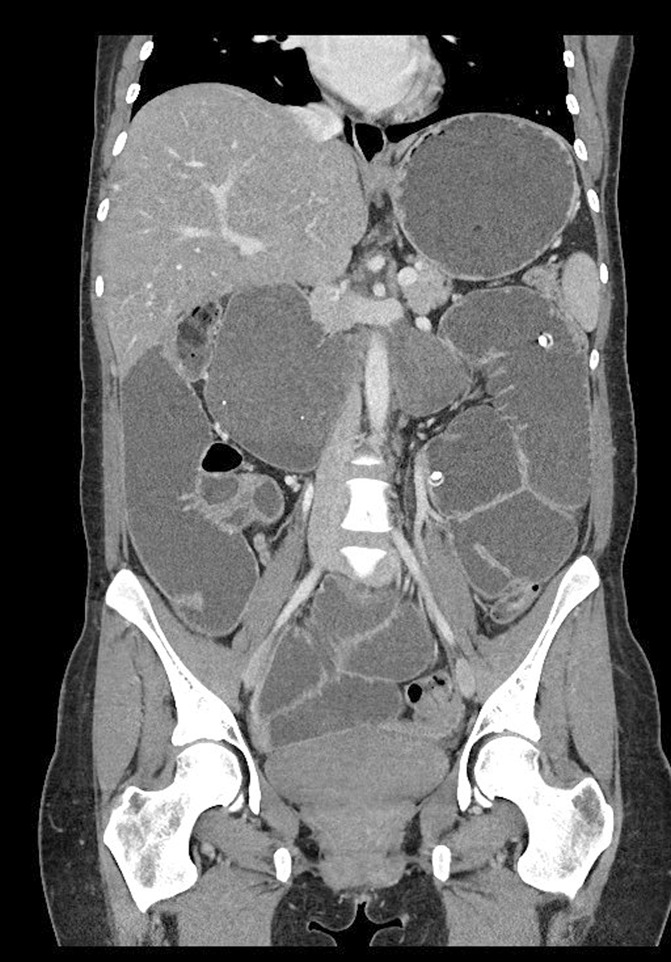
A CT image showing massively dilated small bowel in a patient with SSc. Duodenum dilated up to 8 cm. Features of “hidebound” seen in jejunum. In addition, incidentally seen is a percutaneous jejunostomy tube placed for nutritional support. SSc, systemic sclerosis.
